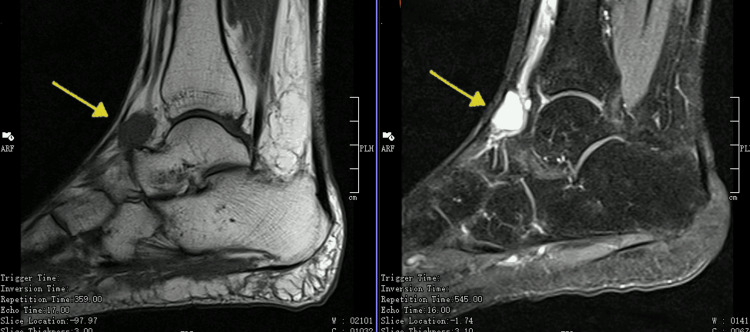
MRI images showing the schwannoma (arrow). The right image is T2-weighted where the schwannoma appears hyperintense, and the image on the left is T1-weighted with the schwannoma appearing isointense MRI: magnetic resonance imaging
